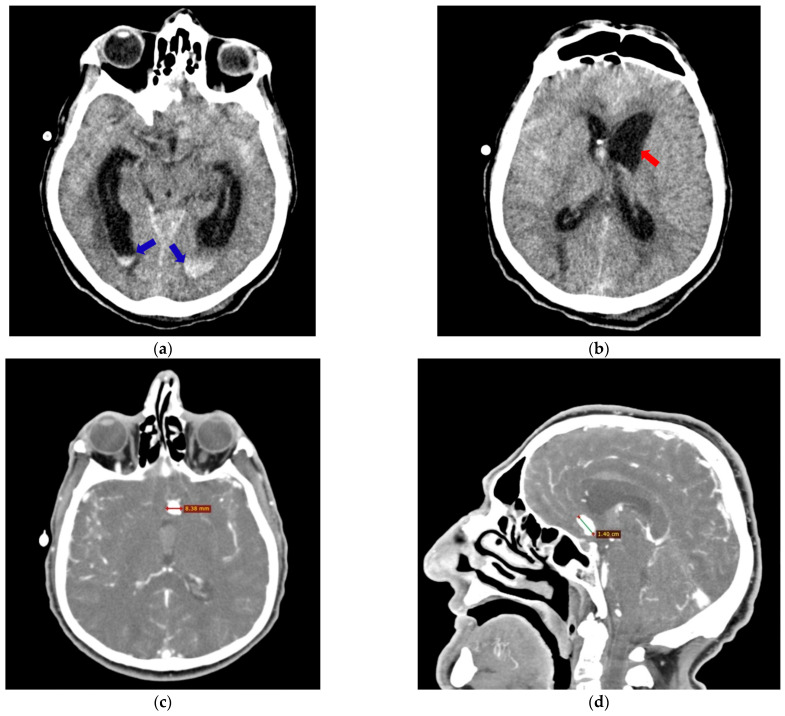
M, 63 years old. Craniocerebral CT revealed a subarachnoid hemorrhage with ventricular flooding (blue arrows), transverse section (a), associated with diffuse cerebral edema with obliteration of the cortical sulci and dilation of the sphenoid horns of the lateral ventricles (red arrow), transverse section (b), imaging features that may suggest a ruptured AcoA aneurysm. CTA identified an aneurysmal dilation of 5.9/8.38/14 mm, possibly at the level of the AcoA; transverse section (c) and sagittal section (d).
